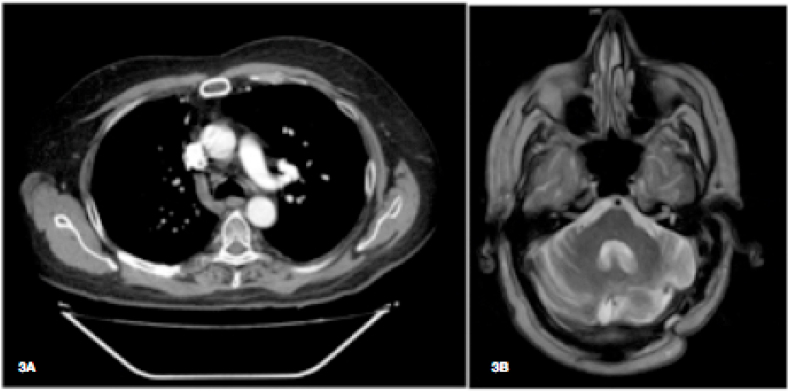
Chest CT scan (3A) and brain magnetic resonance imaging (3B) one year after last surgical procedures.
